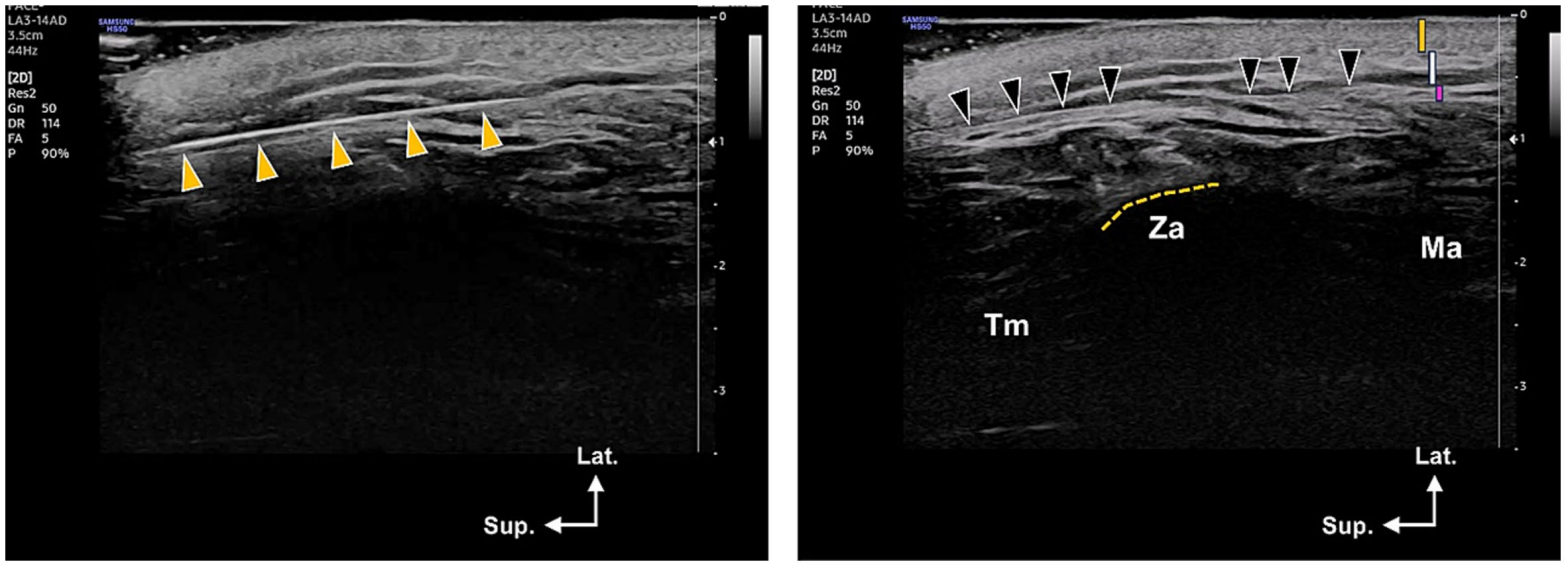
Ultrasonography images showing the location of the thread within the lateral face. The left image indicates the cannula’s location at the temple area, which was taken at the level of the lateral canthus. The yellow arrowheads point to the thread’s position within the temporoparietal or superficial temporal fascia. The right image displays the thread’s location within the subcutaneous layer (indicated by black arrowheads, which refer to the supra-SMAS in this article), specifically the deep portion of the subcutaneous layer just superficial to the SMAS layer. This image was taken at the zygomatic arch (ZA) level. In the upper right corner of the right image, the orange, white, and pink bars represent the skin, subcutaneous, and SMAS layers, respectively. Tm, Temporalis muscle; Ma, masseter muscle; Lat., lateral, Sup., superior.
